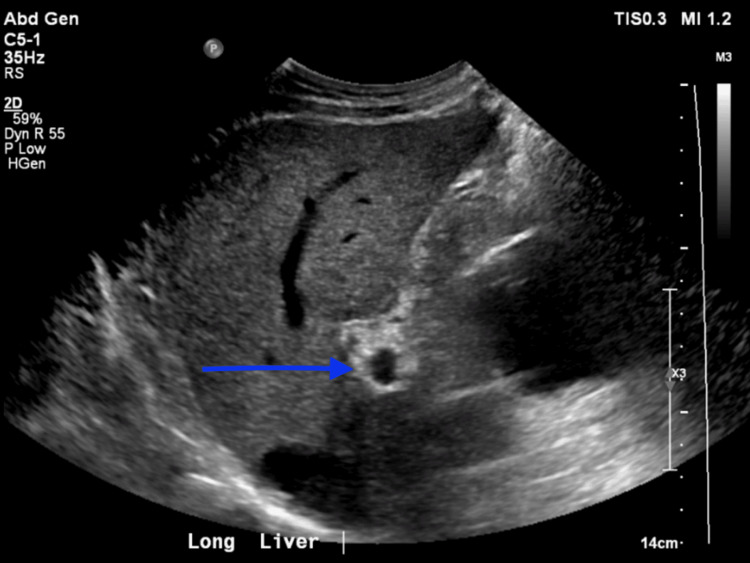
Right upper quadrant ultrasound demonstrating a cystic lesion at the inferior aspect of the right hepatic lobe (subcapsular region). The lesion appears anechoic with well-defined borders and no solid components, initially interpreted as a simple hepatic cyst.
